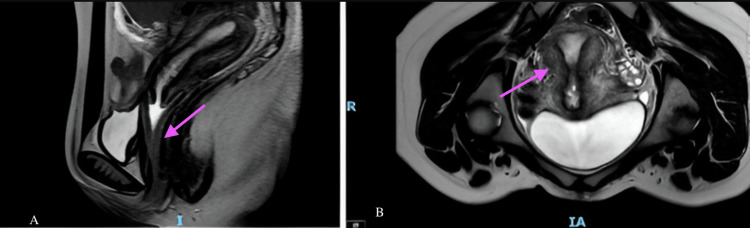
Pelvic MRI Pelvic magnetic resonance imaging (MRI) showing a longitudinal vaginal septum. (A) Sagittal T2-weighted image demonstrating a linear hypointense band (arrow) partially dividing the vaginal canal in the anteroposterior direction. (B) Axial T2-weighted image identifying the septum as a midline hypointense structure (arrow) separating the vaginal lumen into two hemichannels. No abnormalities were observed in the uterus or cervix
